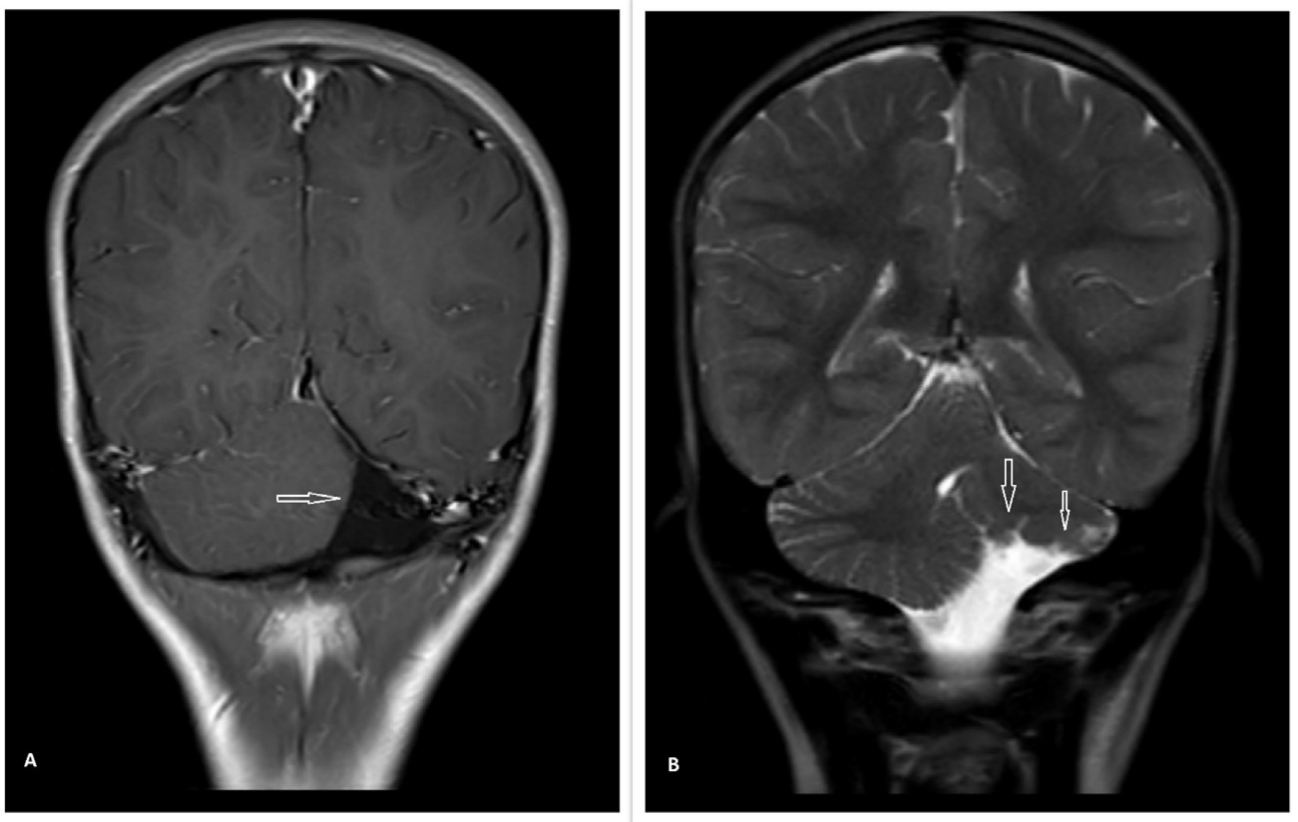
Coronal T1W with GAD (A), T2W (B) sequences brain MRI of left cerebellar hypoplasia. The residual left cerebellum shows normal gray-white matter differentiation and foliation (arrows). The right cerebellar hemisphere shows normal volume and morphology.
